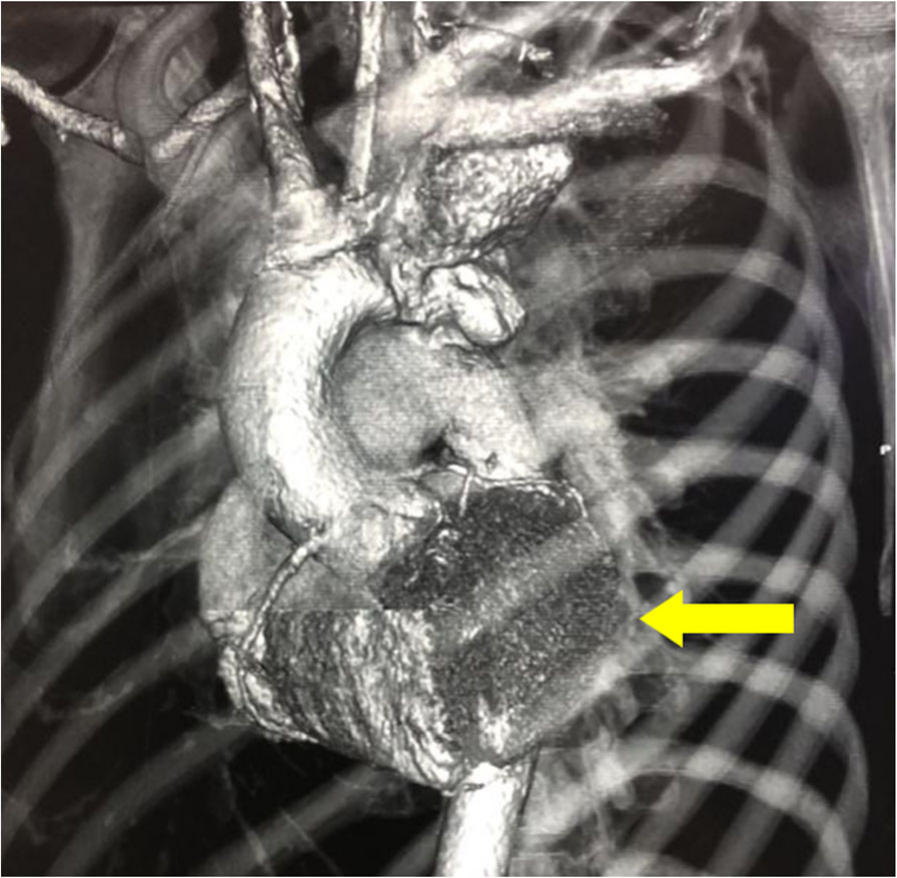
A reconstructed CTA showing the position and relative size of the aneurysm in the descending aorta (yellow arrow) near left subclavian artery
